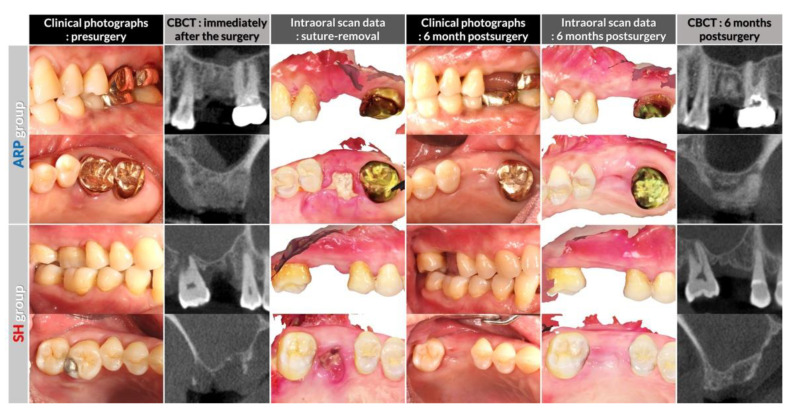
Representative clinical photographs, intraoral scan and conebeam-computed tomography (CBCT) images.
